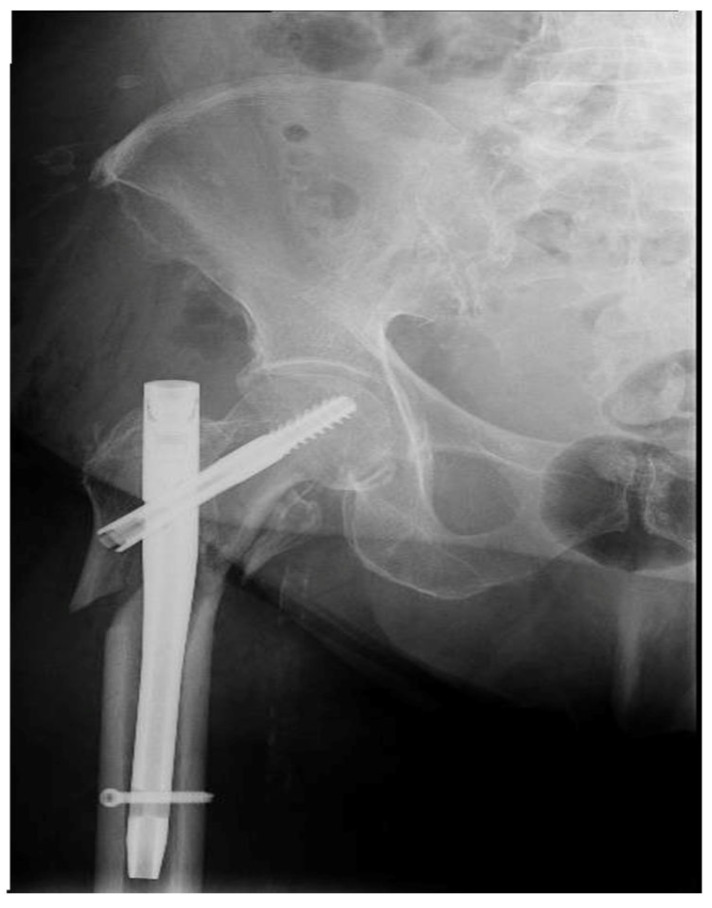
Post surgery X-ray showing the fracture and the position of the implant within the bone.
